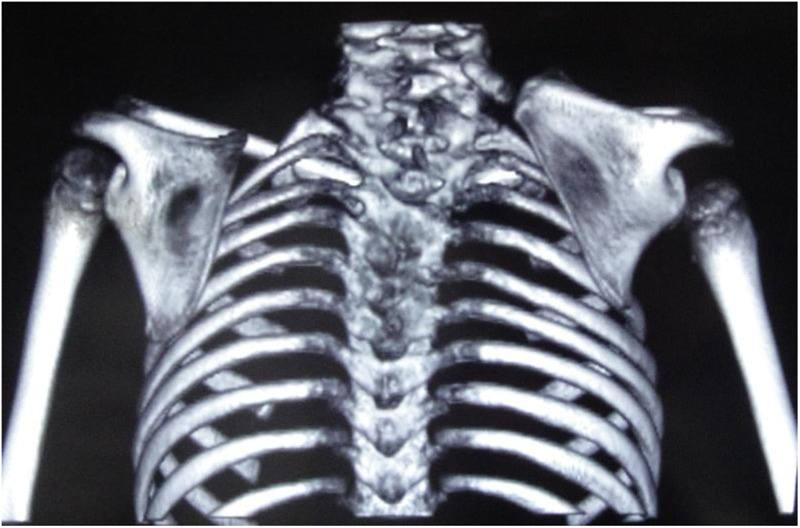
case 6. Preoperative 3D-CT demonstrated a significantly elevated right scapula and an omovertebral bone.
